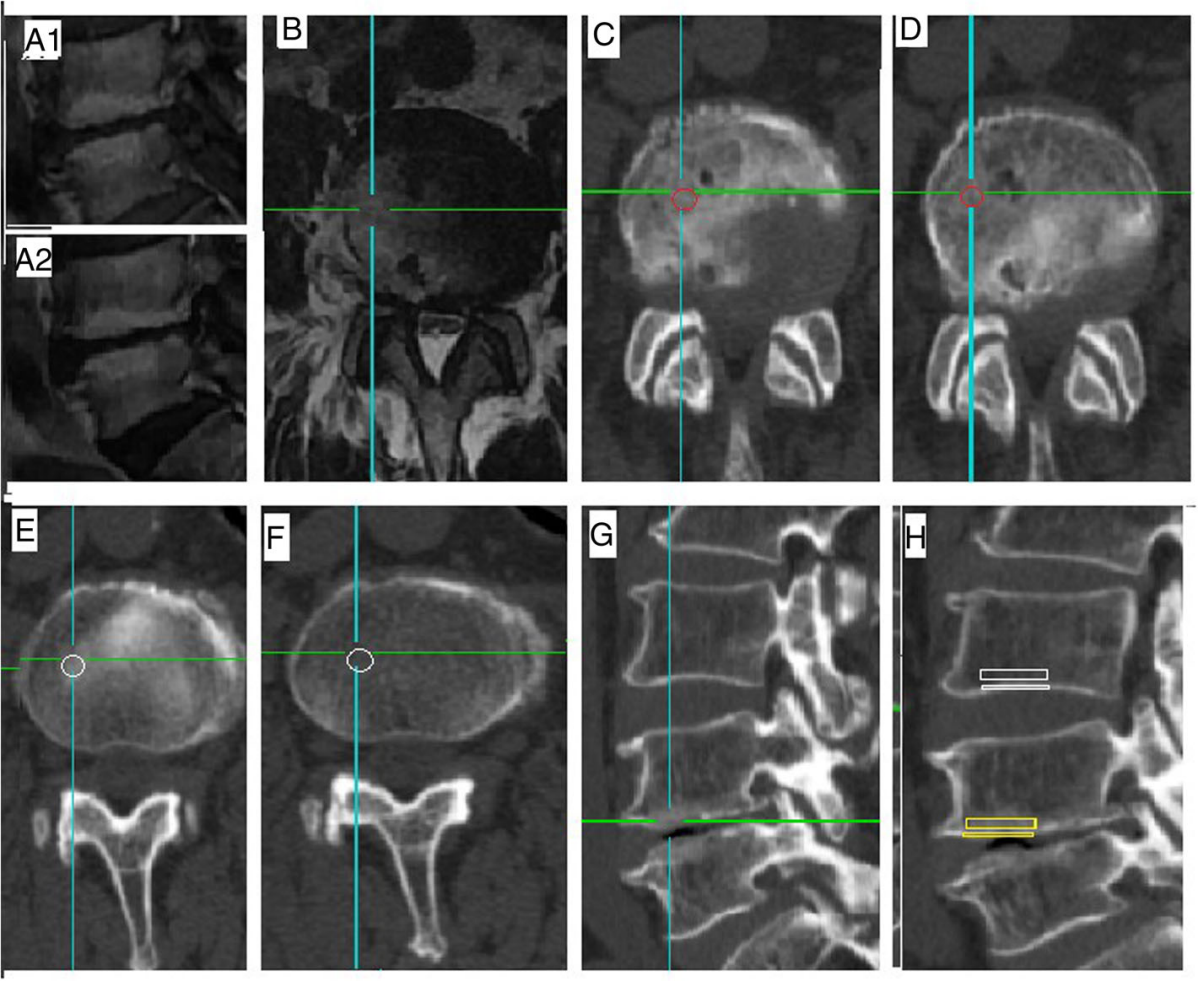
Measurement of HU value. A1/A2, signal changes of endplate and bone marrow on MRI scans (corresponding T1W/T2W); B, locate MCs regions in axial plane; C/D, oval ROI of HU value in axial plane with MCs (corresponding endplate/bone marrow); E/F, oval ROI of adjacent vertebra without MCs (corresponding endplate/bone marrow); G, located MCs regions in sagittal CT plane; H, rectangular ROI in sagittal CT plane (small yellow rectangle showed endplate with MCs, big yellow rectangle showed bone marrow with MCs; small white rectangle showed endplate with no MCs, big white rectangle showed bone marrow with no MCs)
